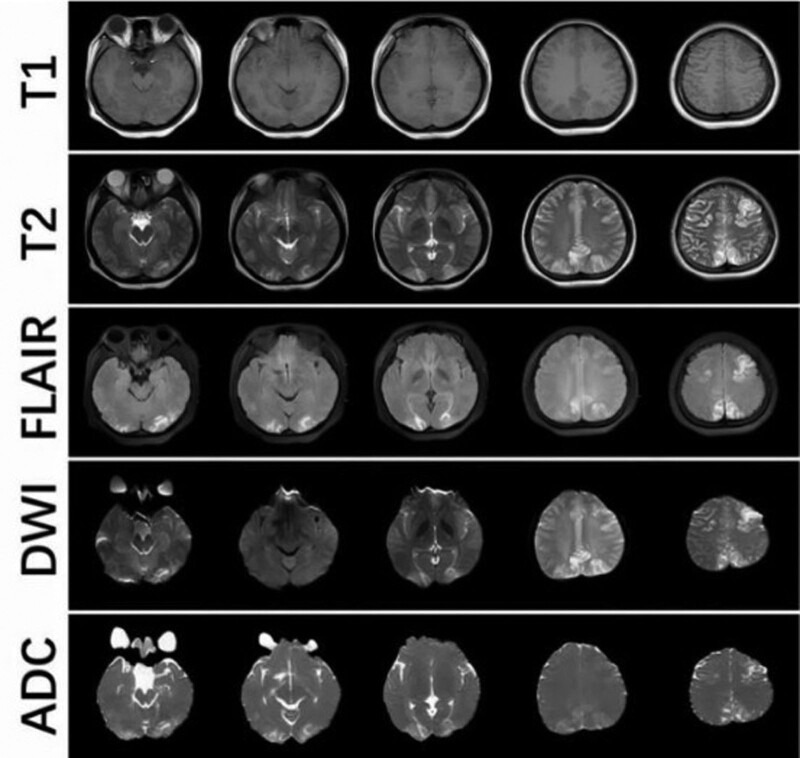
The patient’s first head MRI showed bilateral long T1-weighted and long T2-weighted signal lesions in the occipital, frontal, and parietal cortices, which were more evident on fluid-attenuated inversion recovery (FLAIR) sequences. On DWI and ADC maps. The lesions had slightly higher signal intensities. These lesions had no effect on the enhancement of scans (not included). ADC = apparent diffusion coefficient, DWI = diffusion weighted imaging, MRI = magnetic resonance imaging.
